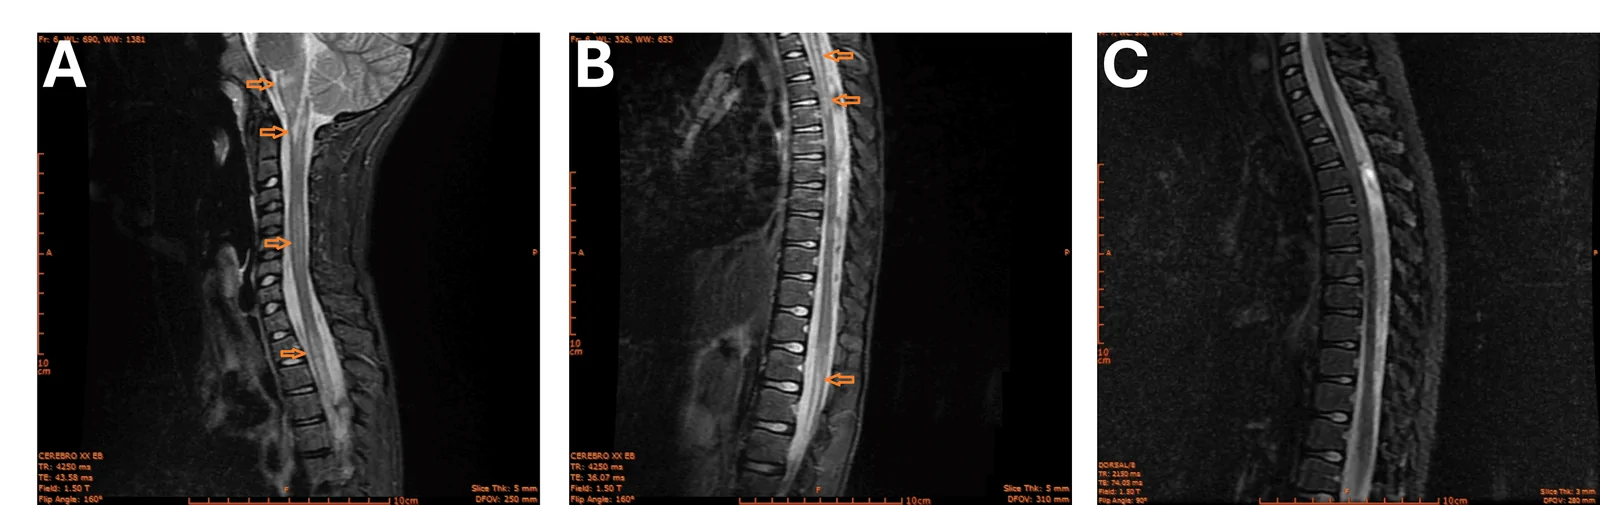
STIR MRI sequence (A) and (B) Baseline images. Hyperintensities are observed (arrows) in the medulla, periaqueductal area, and area postrema, along with involvement of the cervical and thoracic spinal cord (centromedullary). In panel B, an additional arrow identifies a hyperintensity extending into the conus medullaris. (C) Four-month follow-up. No spinal cord abnormalities are present. STIR: Short tau inversion recovery. A magnetic resonance imaging (MRI) technique that suppresses fat signals, enhancing the visualization of tissues and pathologies.
